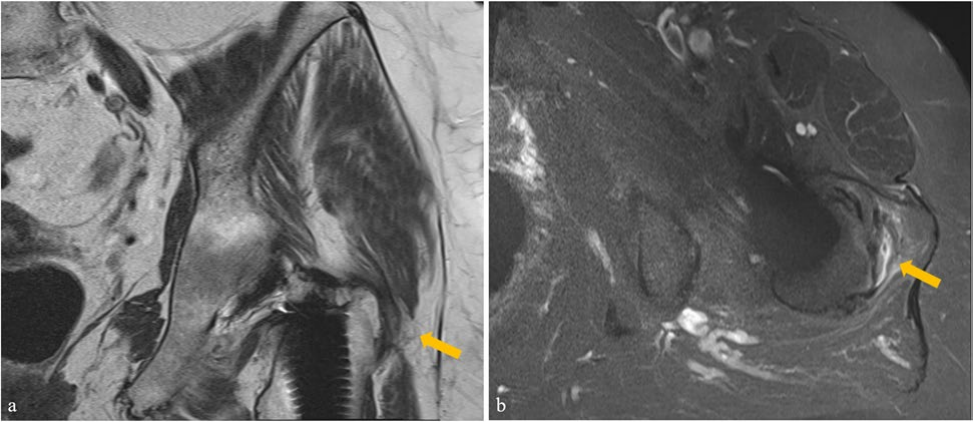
A 69-year-old female patient admitted for lateral hip pain following THA with clinical suspicion of GTPS. Coronal T1-weighted image (a) depicting partial detachment of the gluteus medius tendon at its insertion (arrow). Axial STIR image (b) reveals a fluid-filled trochanteric bursa with perifocal soft-tissue edema (arrow). GTPS, greater trochanteric pain syndrome; STIR, short-tau inversion recovery; THA, total hip arthroplasty
